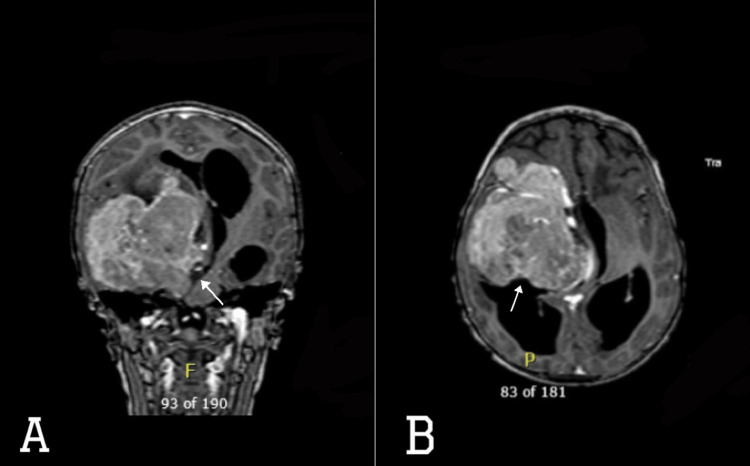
MRI brain with contrast (A) axial and (B) coronal views showing large lobulated right supratentorial frontotemporal mass with heterogeneous enhancement (white-filled arrows) on post-contrast images. It measures 6.7 x 7.5 x 7.1 cm (TR x AP x CC), causing significant mass effect on and displacement of the right lateral ventricle frontal and temporal horns, third ventricle, right basal ganglia, right cerebral peduncle, midbrain, pons, deep cerebral veins, right carotid terminus, right MCA, and right PCA. There is severe supratentorial hydrocephalus with CSF permeation (transependymal edema), 0.9 cm leftward midline shift, and right arteral downward trans-tentorial herniation MCA - middle cerebral artery, PCA - posterior cerebral artery; CSF - cerebrospinal fluid
